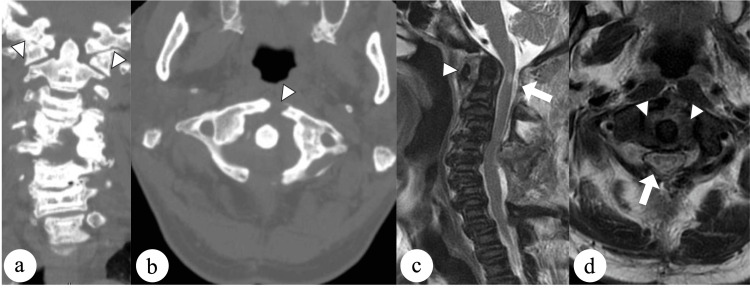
Radiological findings at one week after surgery. (a) Coronal and (b) axial computed tomography scans showing a displaced fracture of the anterior arch with widening of less than 7 mm (arrowheads). (c) Sagittal and (d) axial magnetic resonance images showing hemorrhage around the fracture site with no injury to the transverse atlantal ligament (arrowheads). Adequate direct decompression was obtained by C1 laminectomy (arrows).
